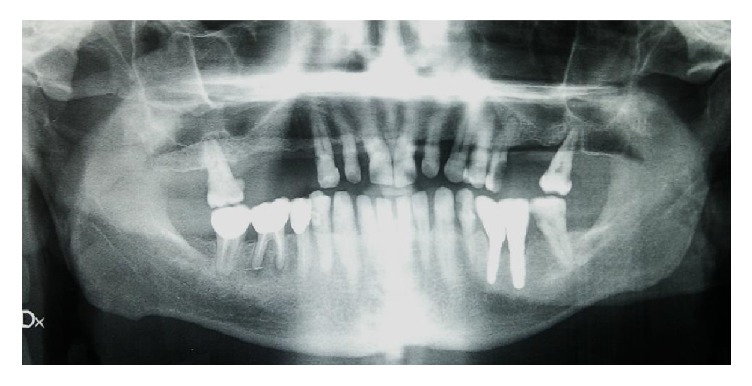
Starting case, full-mouth intraoral radiographic exam.
